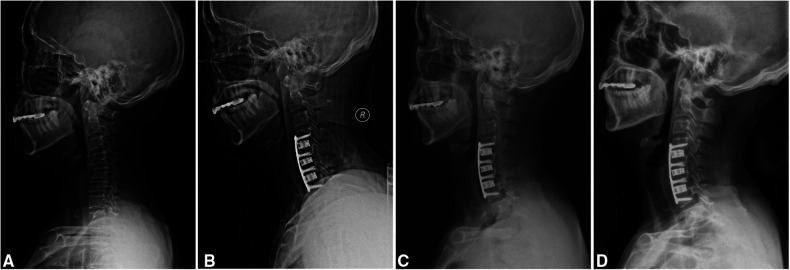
A 60-year-old woman with degenerative cervical spondylosis. (A) A preoperatively lateral radiograph in a neutral position. (B) This patient was treated with 3-level anterior cervical discectomy and fusion using a 3D-printed titanium cage (C4-7). (C) A radiograph at three months post-operatively shows that the implant is in a good position. (D) A radiograph at the latest follow-up shows a satisfactory outcome.
